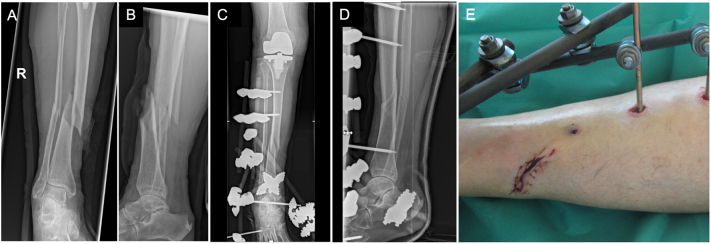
Anterior-posterior (A) and lateral (B) X-ray of the distal spiral tibia shaft fracture (AO type 42-A1) with concomitant fibula shaft fracture of the right leg. Postoperative anterior-posterior X-ray after closed reduction and external fixation (C). Photograph of the primarily sutured wound with a length of 5 cm directly over the fracture site (D).
